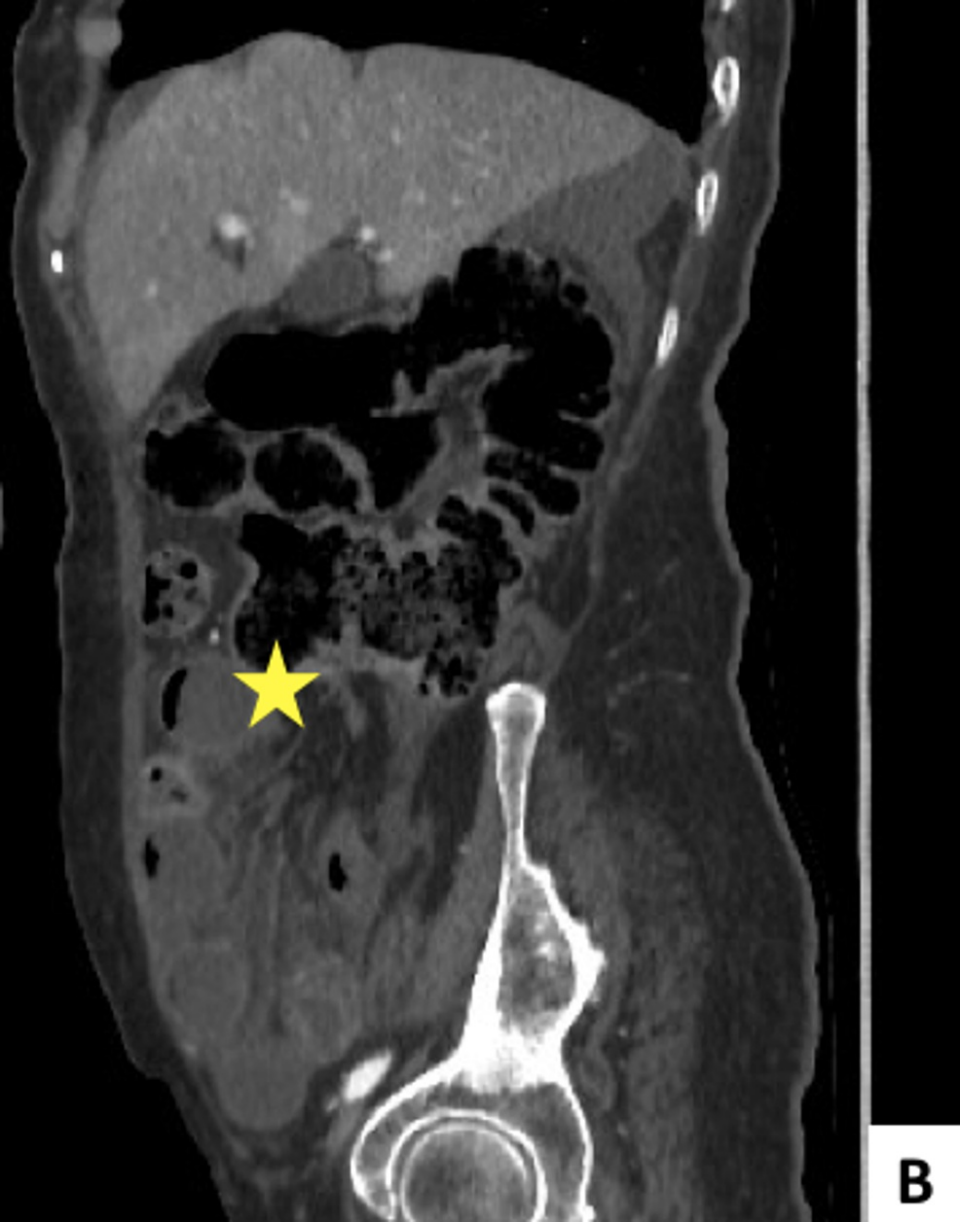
Sagittal (B) CT scans demonstrating strangulated small-bowel loops in a “sac-like” formation, shifting upward the ascending colon (yellow star).
